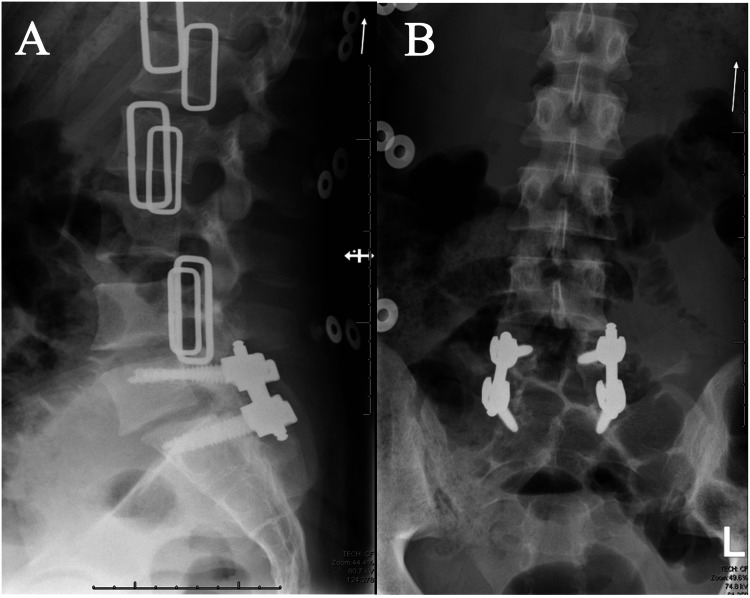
Post-operative anteroposterior and lateral lumbar spine x-rays (A) Lateral x-ray of the lumbar spine showing near anatomical post-operative reduction of the L5/S1 traumatic dislocation and spondylolisthesis with L5/S1 posterior-lateral pedicle screw instrumented fusion and (B) anteroposterior x-ray of the lumbar spine showing successful L5/S1 posterior-lateral pedicle screw instrumented fusion.
